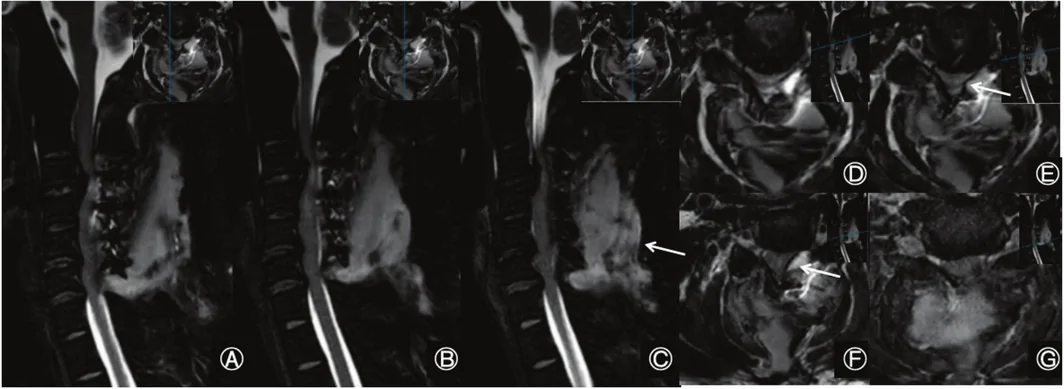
An emergent MRI on postoperative Day 7 revealed a large hematoma within the paraspinal muscles posterior to the C2–C5 laminae, compressing the spinal cord.
